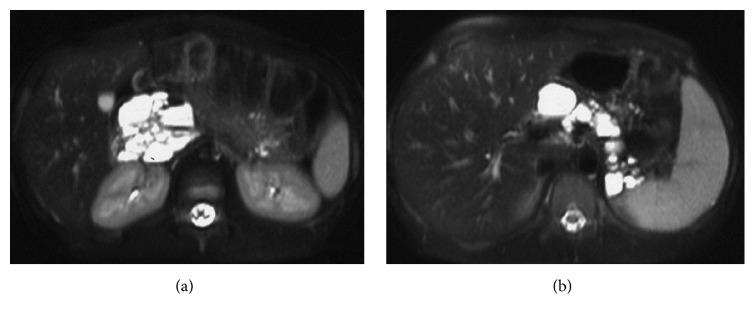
(a) T2-weighted fat-saturated transverse MR image obtained at the level of the pancreatic head shows multiple, high signal cysts of varying size in the expected region of the head of the pancreas without identifiable pancreatic tissue. (b) T2-weighted fat-saturated transverse MR image obtained at the level of the body and tail of the pancreas reveals complete replacement by multiple, bright-signal cystic masses of varying size with thin and smooth walls.
